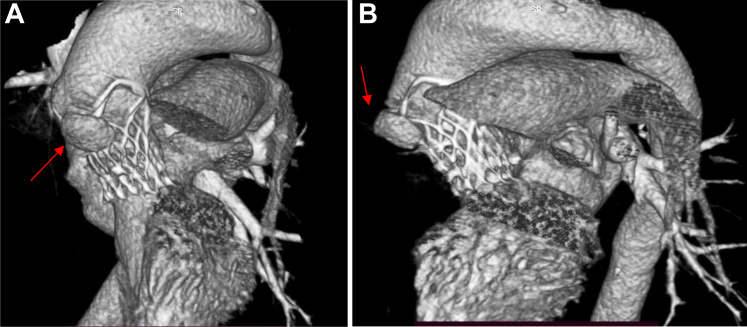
CT 3D Reconstruction of Aortic Root With the Prosthetic Valve (A) Perivalvular mycotic aneurysm seen in aorta through the upper edge of valve (enface view). (B) Perivalvular mycotic aneurysm with a small neck (profile view); red arrow towards the aneurysm, yellow towards the upper edge of the prosthetic valve. See Video 1.
